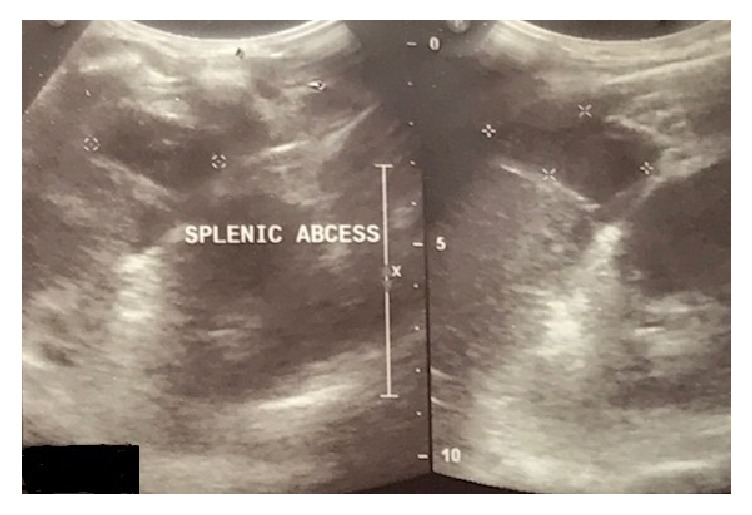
Ultrasound of the abdomen showing multiple hypoechoic splenic and perisplenic collections.
